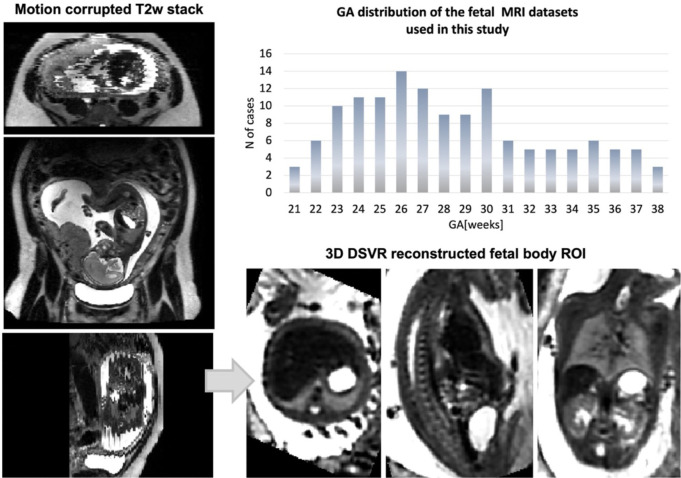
Fetal MRI 3T datasets used in this study: Gestational age distribution and an example of a 3D DSVR reconstructed fetal body.
